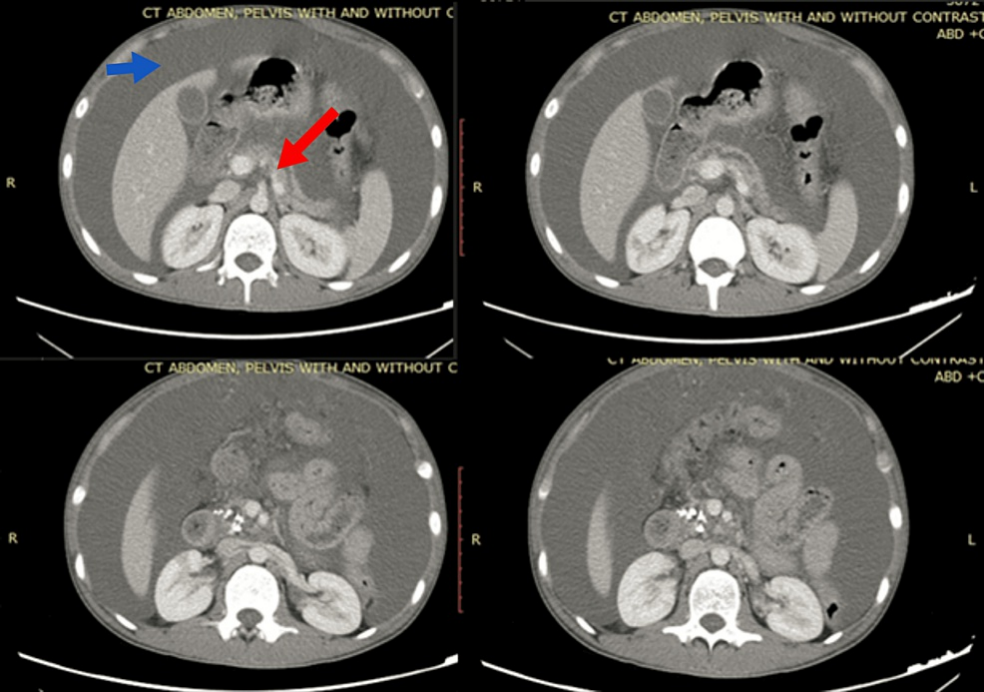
CT of the abdomen and pelvis with contrast The blue arrow showing ascites and the red arrow showing pancreatic calcification.
